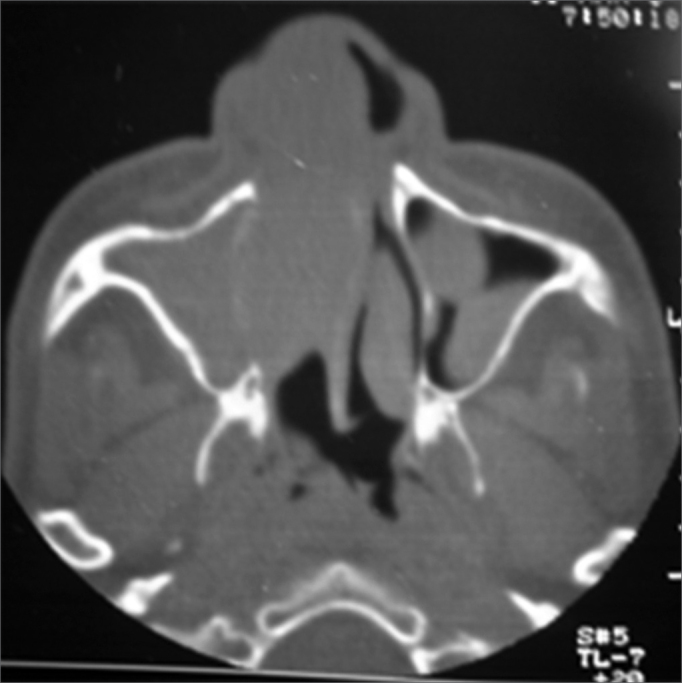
Computed tomography of the paranasal sinuses - axial plane. Soft tissue attenuation material occupies the right nasal fossa, the right maxillary sinus, and part of the left maxillary sinus. The cartilaginous septum is deviated to the left. There is no bone destruction.
